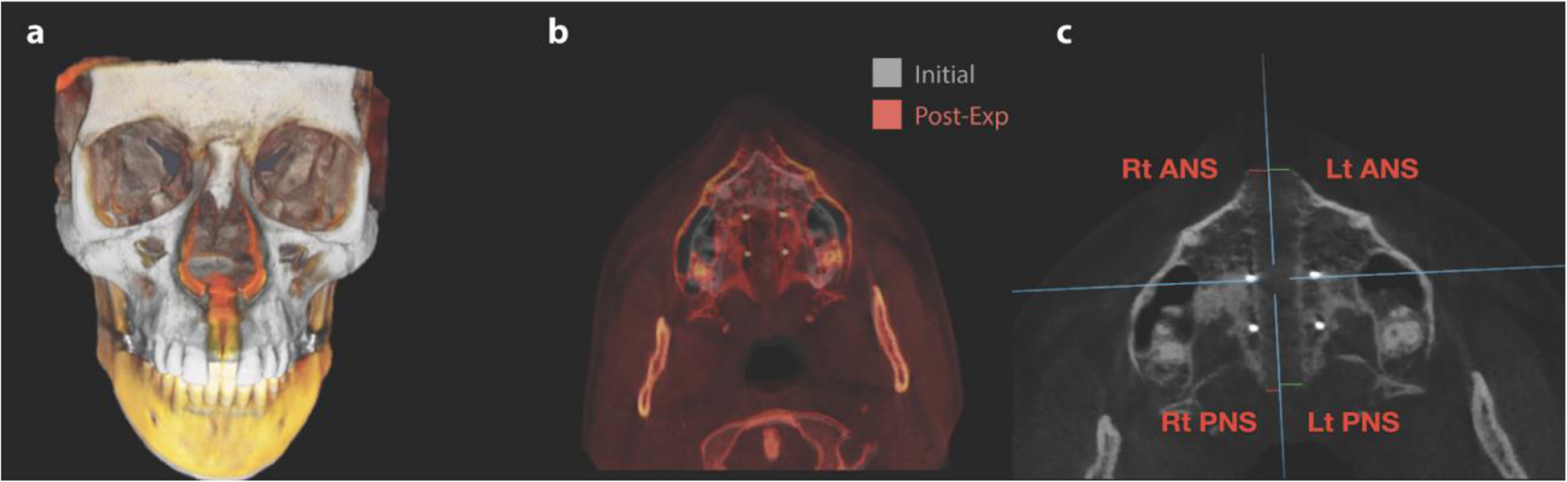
a 3D superimposition of pre- and post-expansion on an individual patient demonstrating changes to mid-face. b Axial view of superimposed pre- and post-expansion. c Axial view of post-expansion CBCT showing measurement from MSP to both the right and left ANS and PNS landmarks with apparent asymmetry
